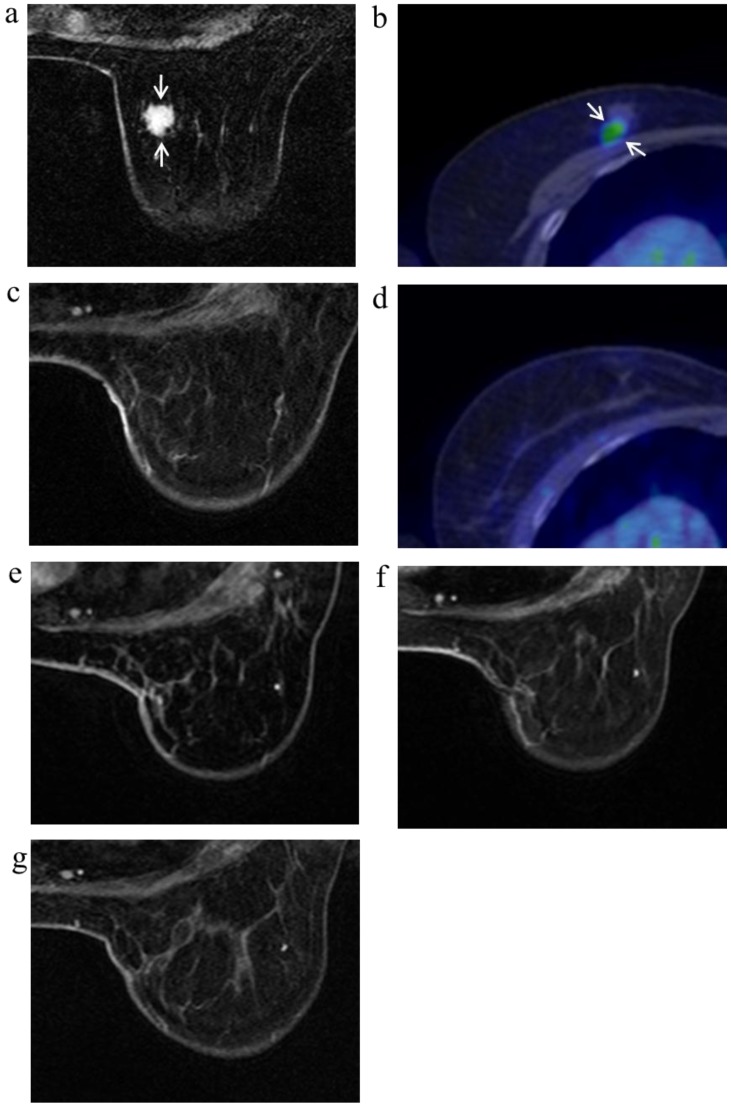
A 63-year-old female (case 8 in Table 1, Table 2 and Table 3) with right breast cancer (cT1cN0M0). CE breast MRI (a) and FDG-PET-CT (b) revealed a breast tumor before KORTUC II therapy (arrows), the region of tumor was 13 mm; After KORTUC II, no recurrence was identified on CE breast MRI and FDG-PET-CT ((c): 10 months; (d): 18 months; (e): 22 months; (f): 34 months; (g): 50 months).
